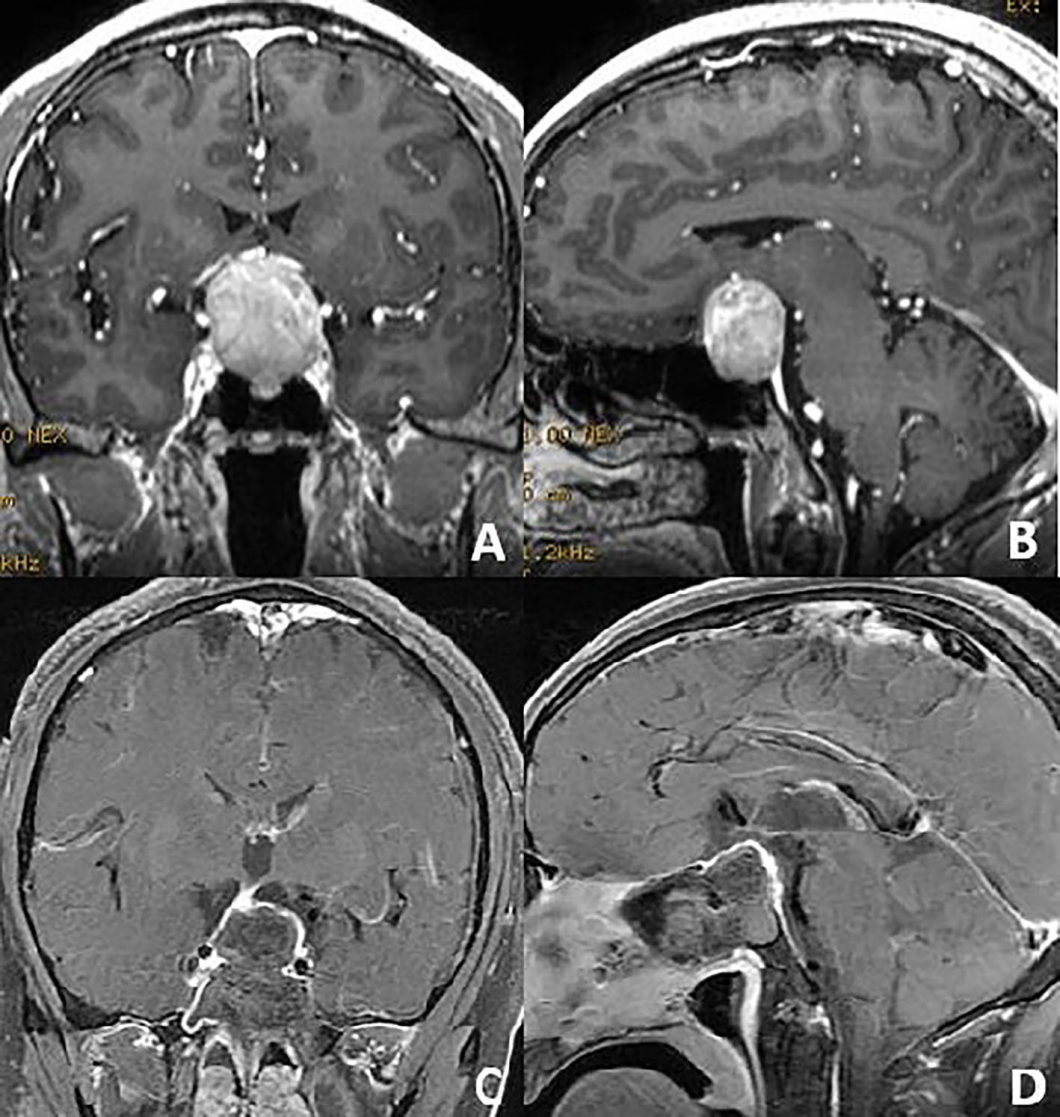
Preoperative coronal and sagittal (A, B) contrast-enhanced magnetic resonance T1-weighted images of a non-functional pituitary macroadenoma. After the endoscopic anterior and posterior pseudocapsule dissection, the adenoma was achieved total resection (C, D).
